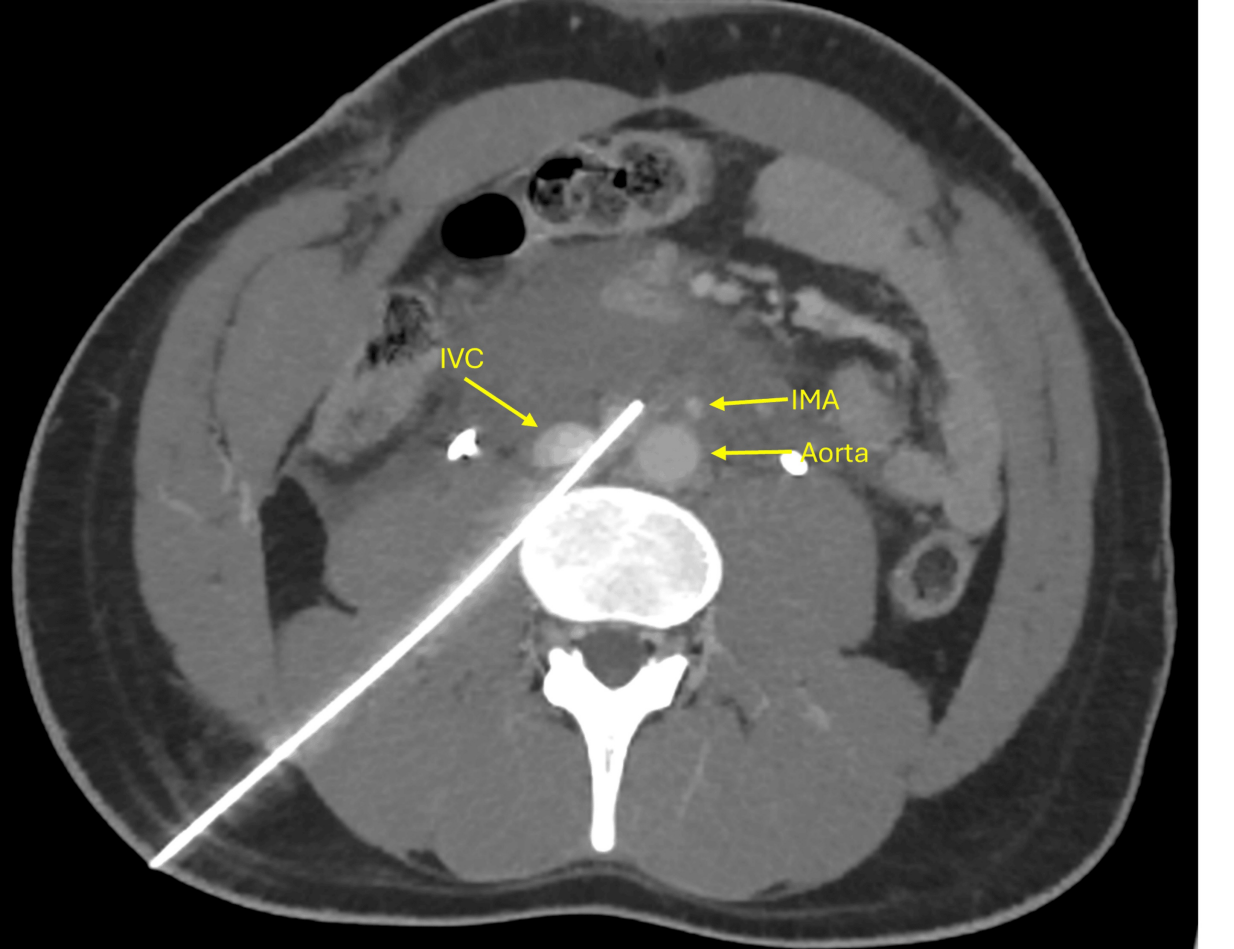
CT of the abdomen showing resolution of the hematoma with patent inferior vena cava (IVC) and inferior mesenteric artery (IMA).
